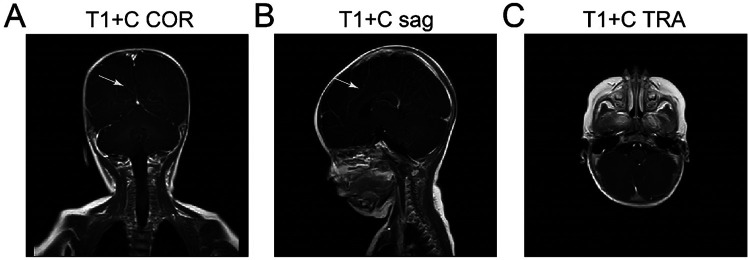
Contrast-enhanced brain MRI on August 26, 2025. (A) Coronal T1-weighted postcontrast image. (B) Sagittal T1-weighted postcontrast image. (C) Axial T1-weighted postcontrast image.
